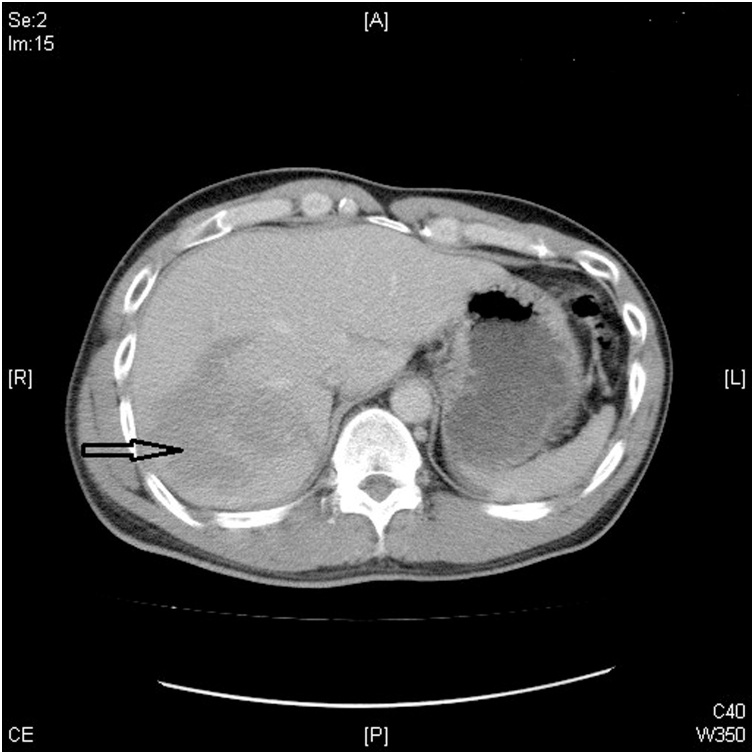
Abdominal computed tomography scan of the 36-year-old man, showing intra-parenchymal laceration with hematoma (arrow).
